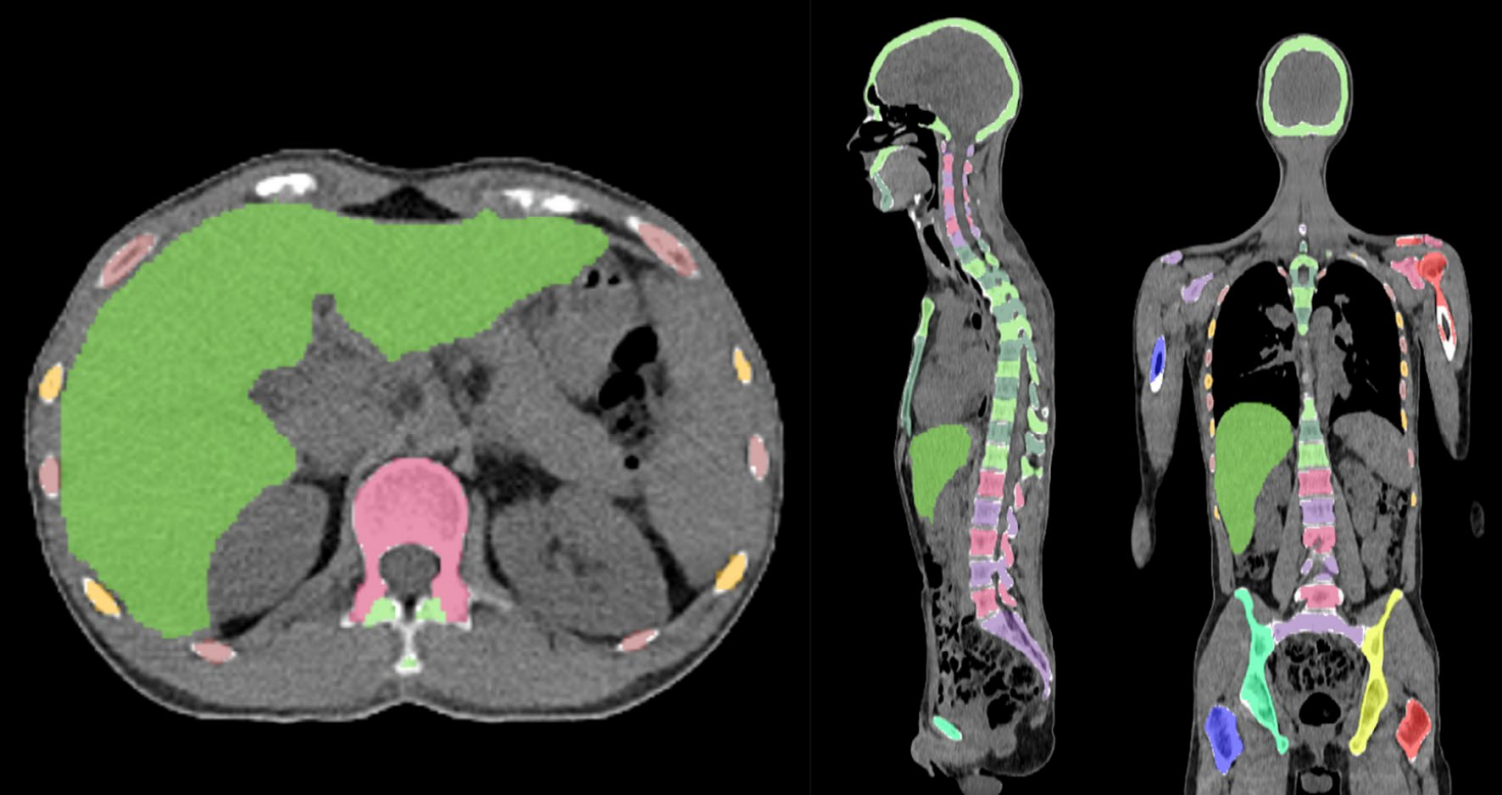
CT: Example of bone and liver segmentations performed by the artificial intelligence-based method.
